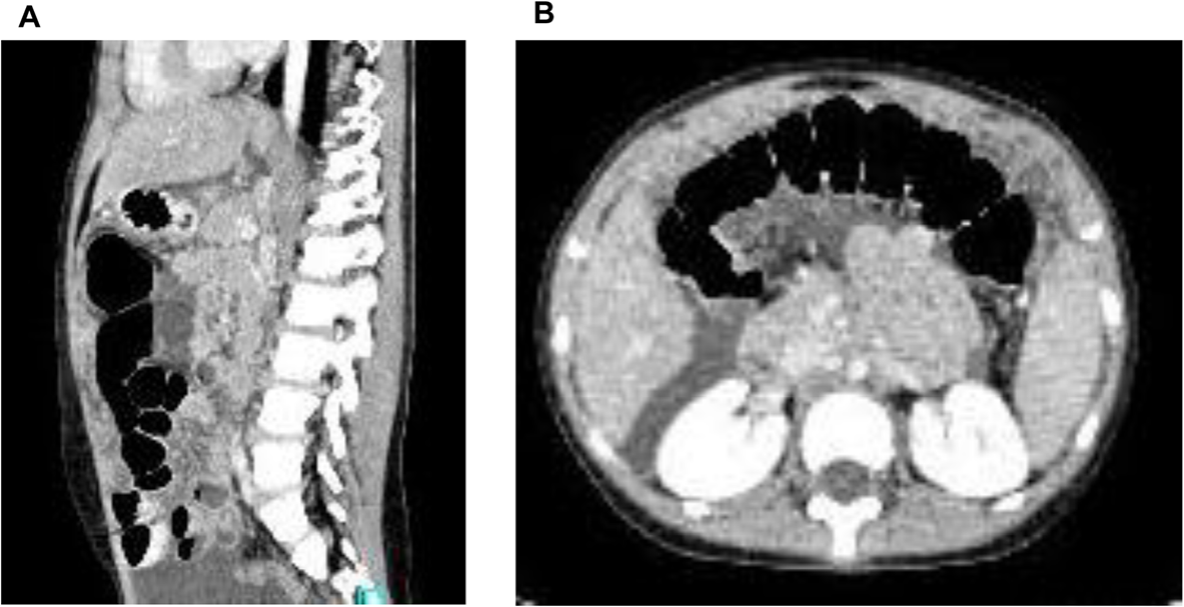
Sagittal (a) and axial (b) computed tomography of the abdomen and pelvis demonstrating diffuse ascites with simmetrical peritoneum thickening, mesenteric adenopathies and nodularity of the omentum with mild bowel wall thickening and “omental cake-like” appearance
